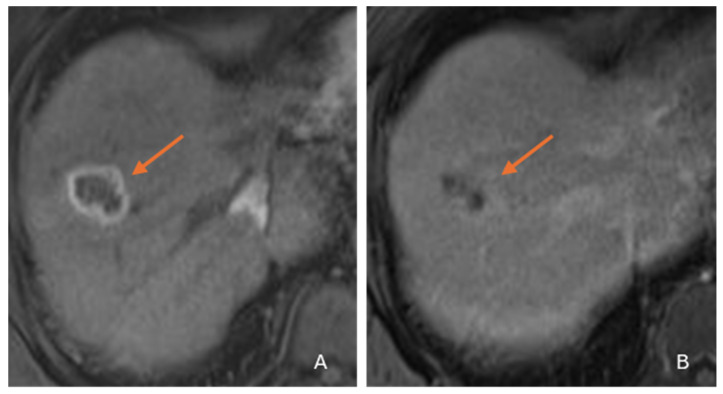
Axial gadolinium-enhanced MRI images in a 55-year-old male with cirrhosis from alcohol use disorder, and a pathologically proven cHCC-CC on biopsy. A 3.1 cm cHCC-CC (arrows) in segment 8 shows LR M features with peripheral rim arterial hyperenhancement (A) followed by central progression on the delayed phase (B).
